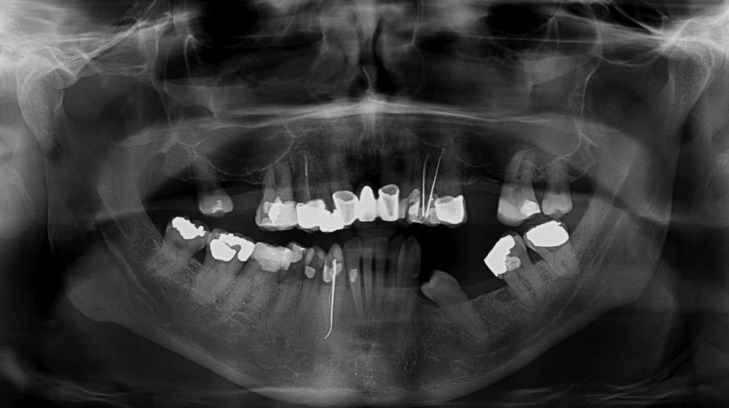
Case 1 - Bilateral bifid mandibular canal in Panoramic radiograph.
